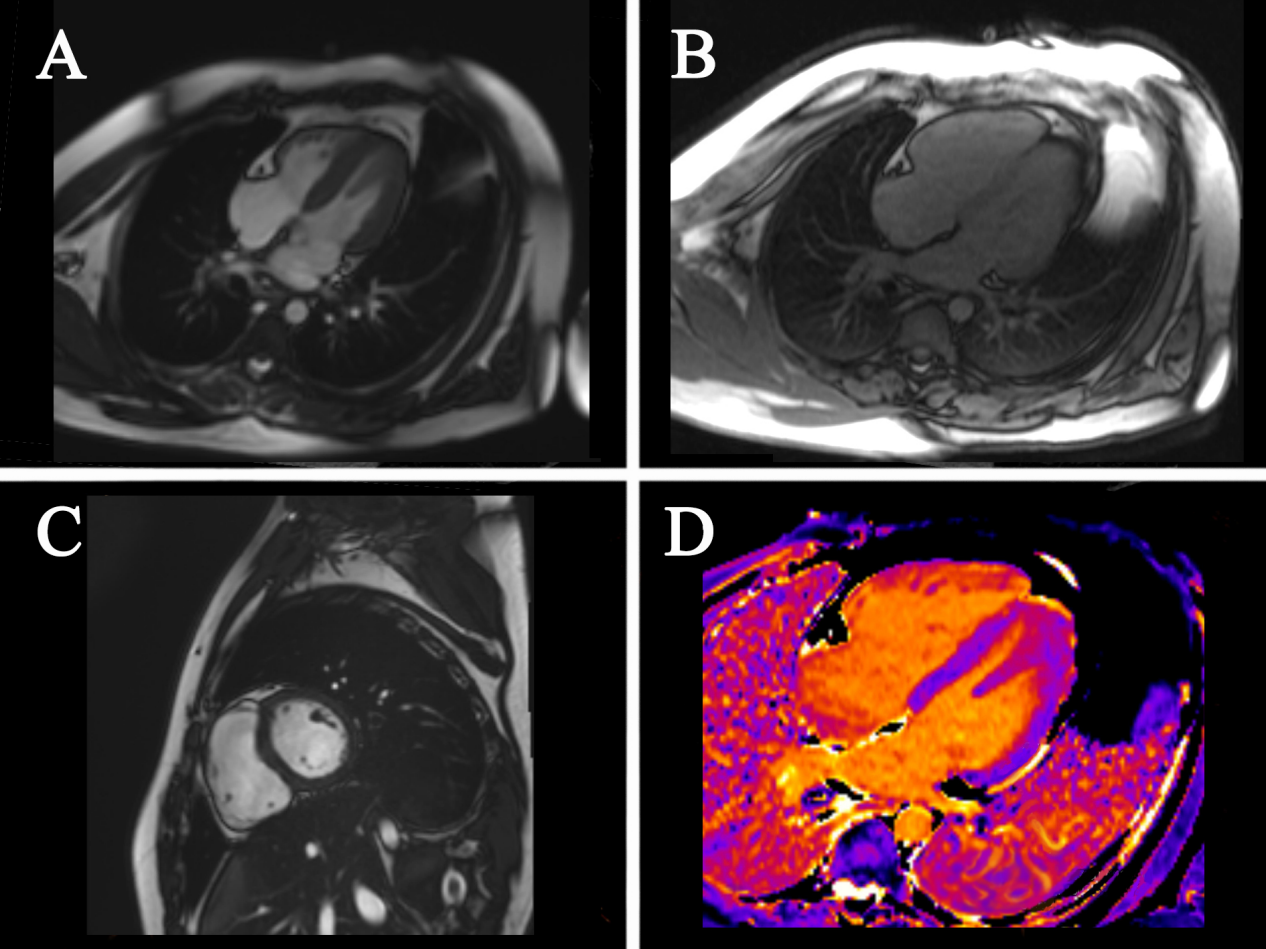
Cardiac MRI images. (A) four-chamber cardiac images; (B)
